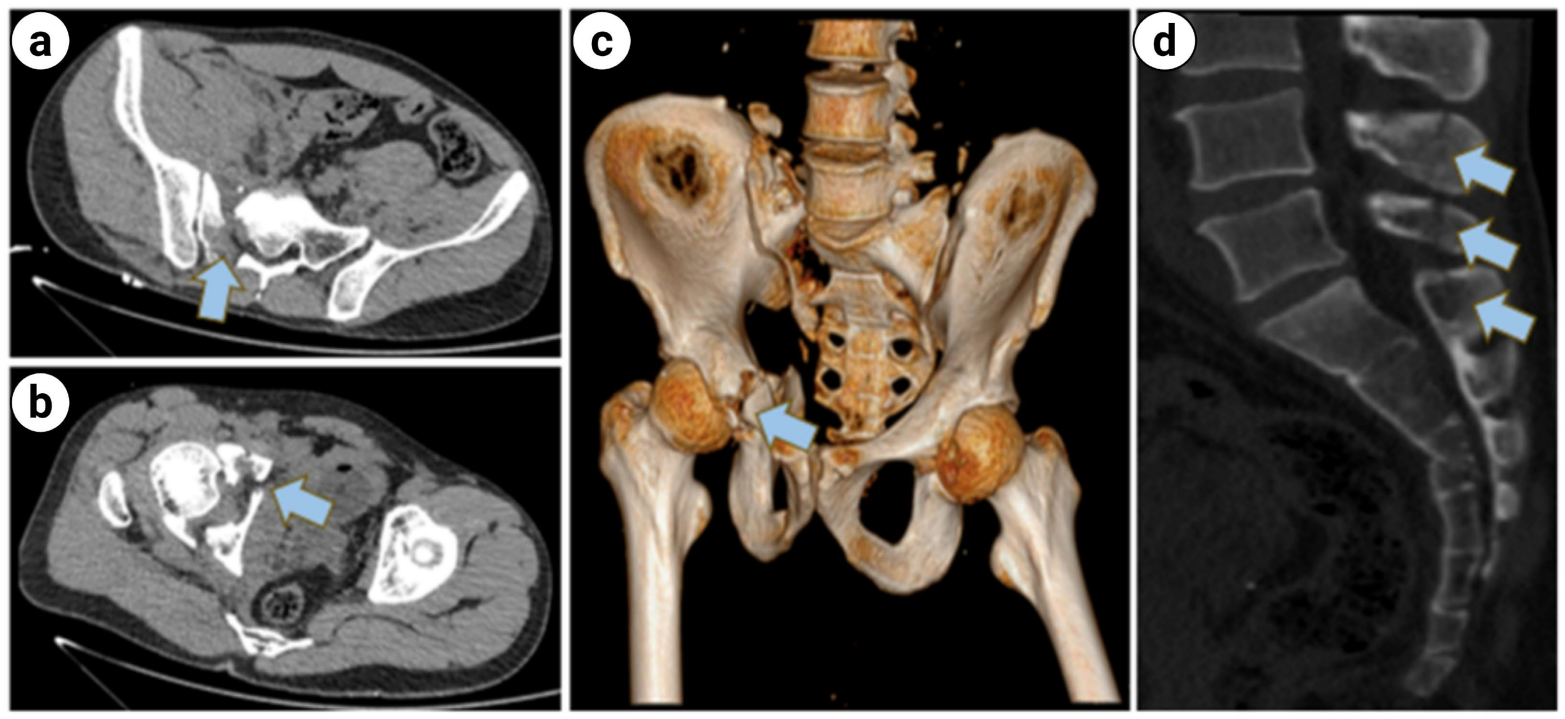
(a,b) Axial CT scan of the pelvis: Fractures of the right sacral ala; (c) 3D CT scan of the pelvis: fracture of the acetabulum and superior pubic ramus; (d) sagittal CT scan of the sacrococcygeal region: The sagittal CT scan of the sacrococcygeal region demonstrates partial fractures of the lumbar spine accessories (indicated by the blue arrows).
